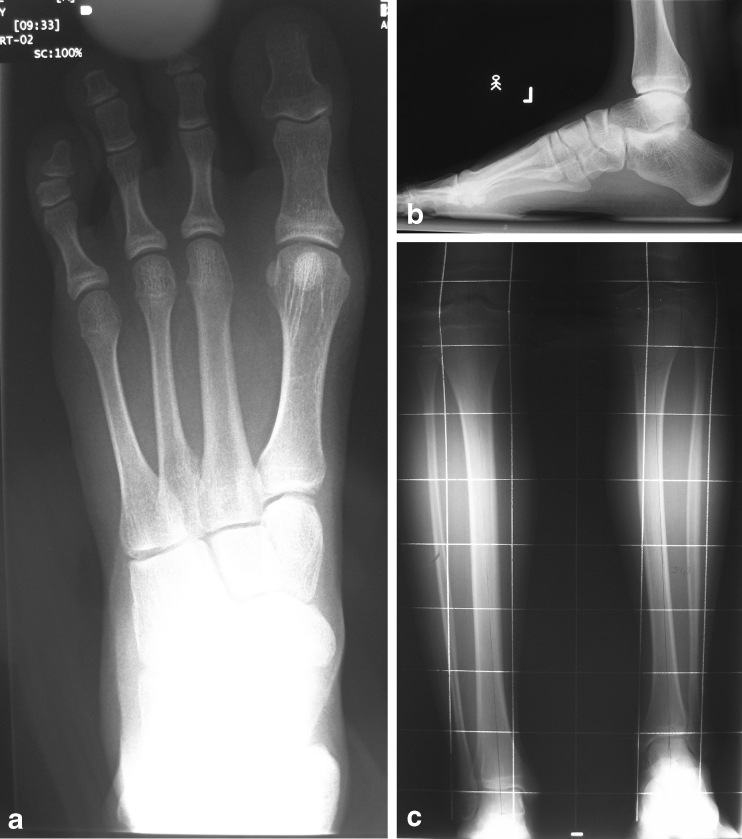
X-ray of left foot hypoplasia with intermediate ray deficiency (a, b, c)
